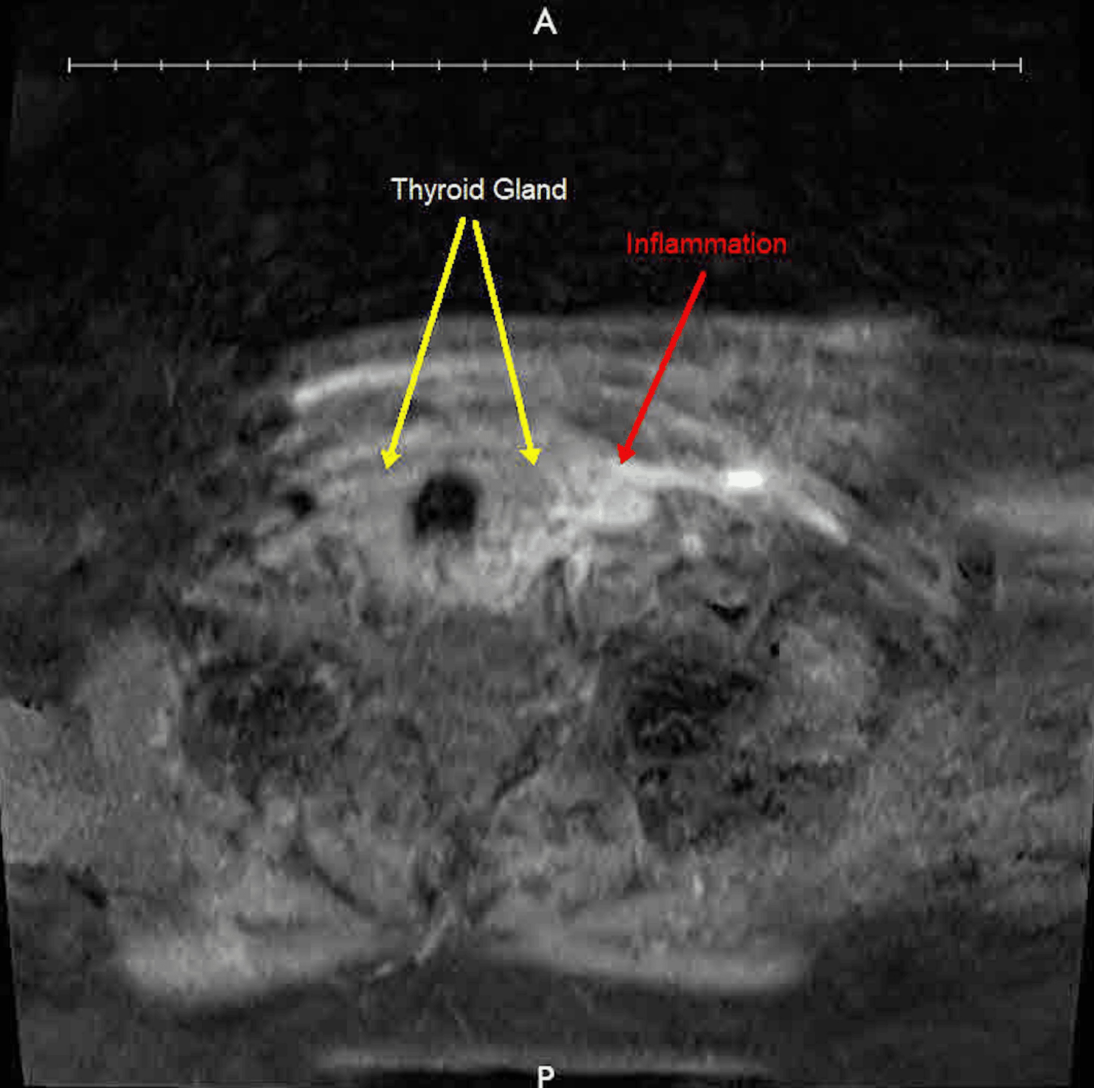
Magnetic resonance imaging (MRI) of the neck SCM: Sternocleidomastoid muscle Neck MRI without contrast showing an inflammatory reaction involving the left SCM and the neck soft tissues to the left of the thyroid gland (yellow arrows) with minimal fluid, but no drainable mature abscess, as indicated by the red arrow
